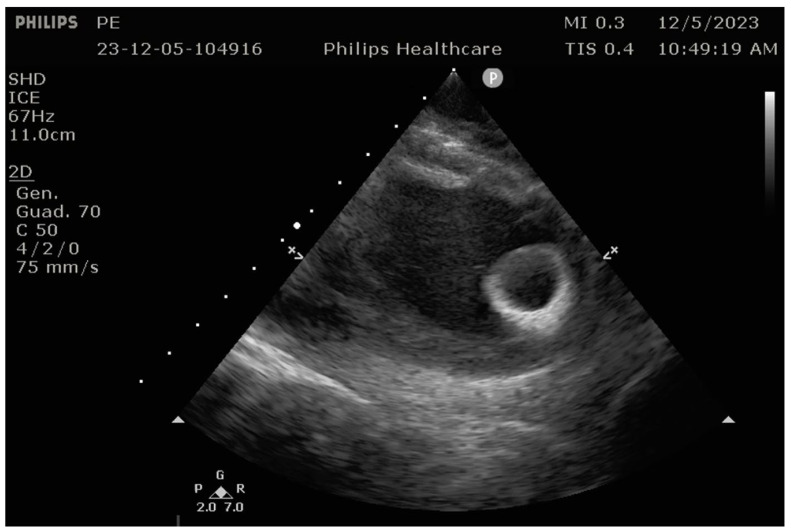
Intracardiac echocardiography during ventricular tachycardia ablation in a patient with LVAD. The apical inflow cannula is well evident.
